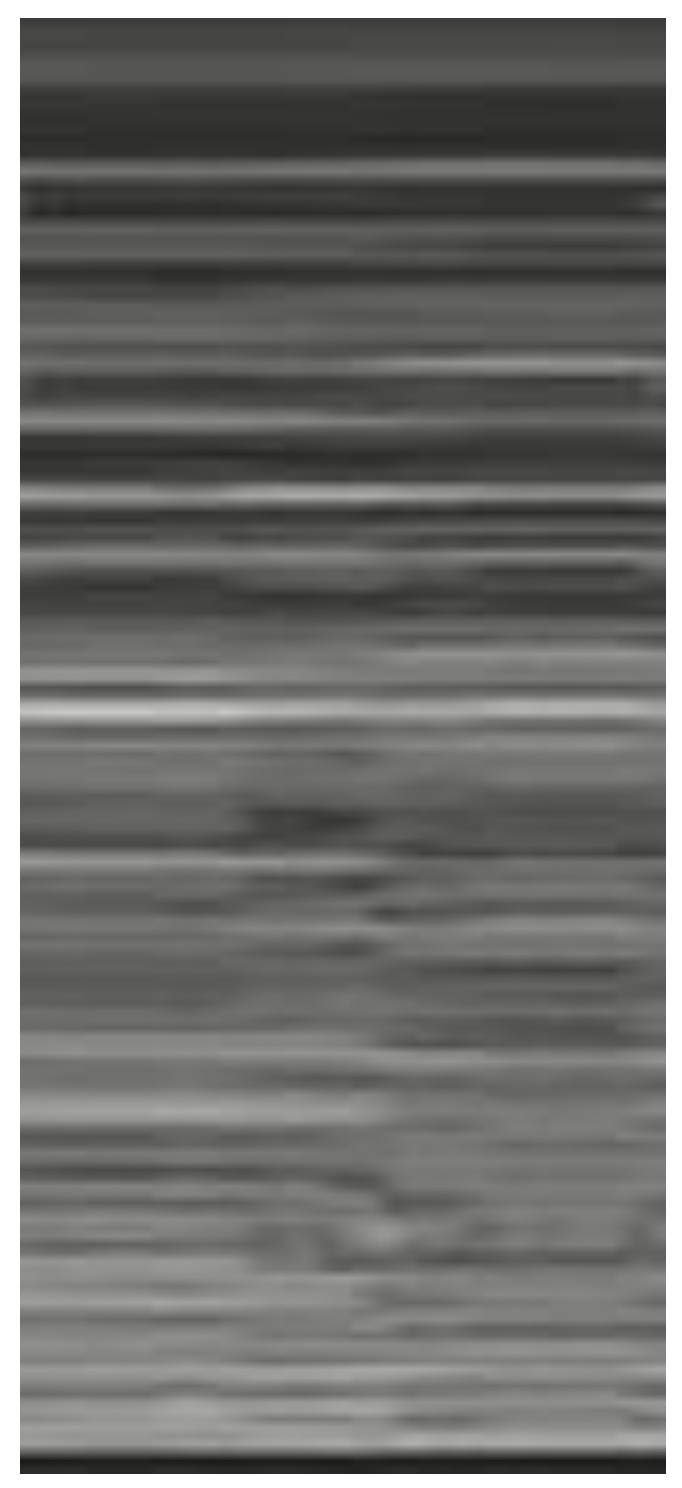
Lung ultrasonography, signs in motion mode (M-mode): barcode sign (sign of the stratosphere), no evidence of pleural sliding, a sign suggestive of pneumothorax.
